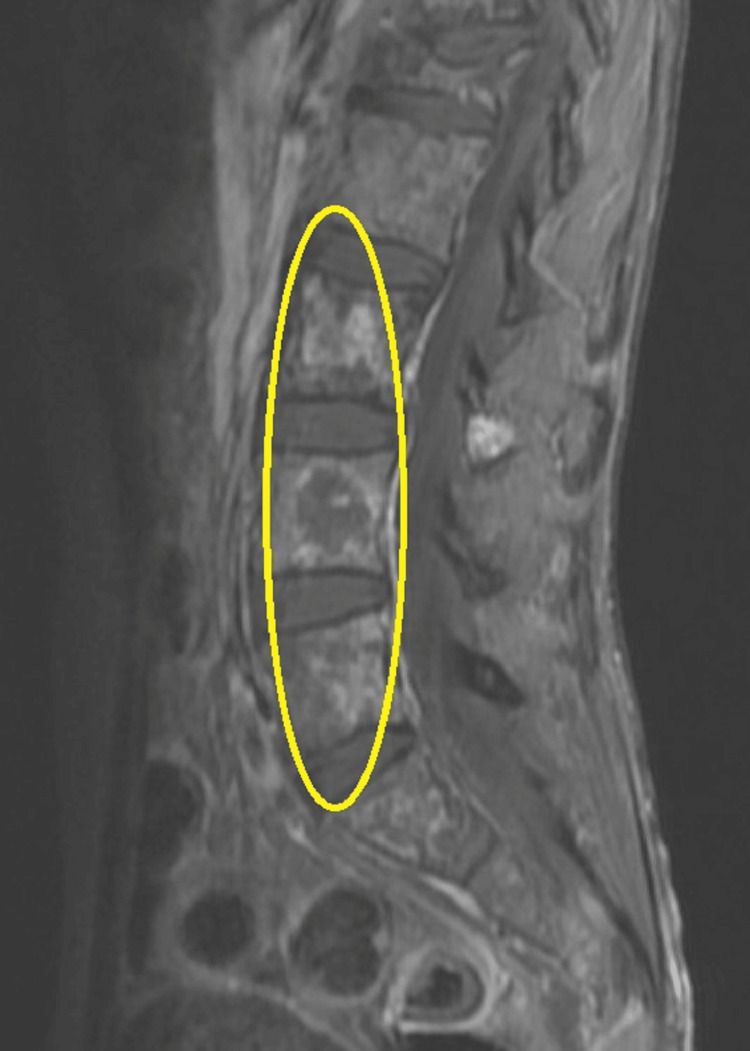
MRI of the abdomen/sagittal section of the lumbosacral region MRI of the abdomen/sagittal section demonstrating destructive bony metastasis involving the lower lumbar vertebrae (L3-L5) (yellow circle).
